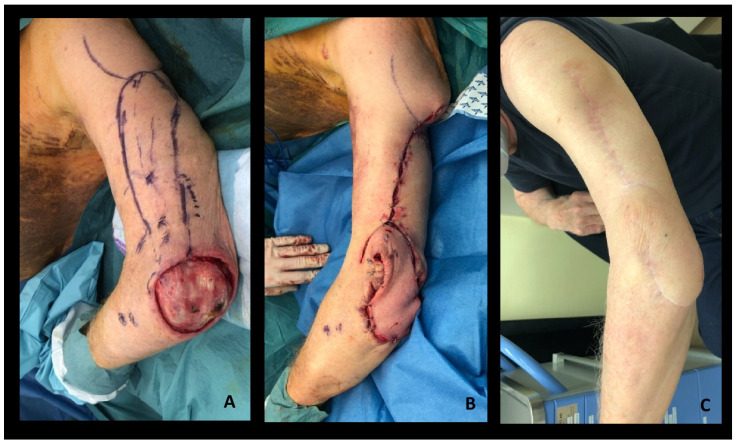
A 77-year-old man underwent excision of a stage III Merkel cell carcinoma involving the posterior aspect of the elbow. A wide local excision left a defect of 10 × 6 cm, with exposure of the olecranon bone. The perforators of the radial collateral artery perforator (RCAP) were found with the handheld Doppler. The skin paddle was designed, including the perforators. (A) The axial flap length was measured to be at least equal to the length from the pivot point to the edge of the defect. Dissection was performed in a subfascial plane and the course of the more distal reliable perforator was followed, up to its origin from the posterior radial collateral artery (PRCA). A skin bridge over the pedicle at the distal margin of the flap was maintained to improve the venous drainage (propeller plus), and the flap was rotated 180° into the defect. (B) The donor site was closed primarily. Postoperative radiotherapy and immunotherapy were performed. The patient had no complications and no movement limitations at a 25-month follow-up (C).
